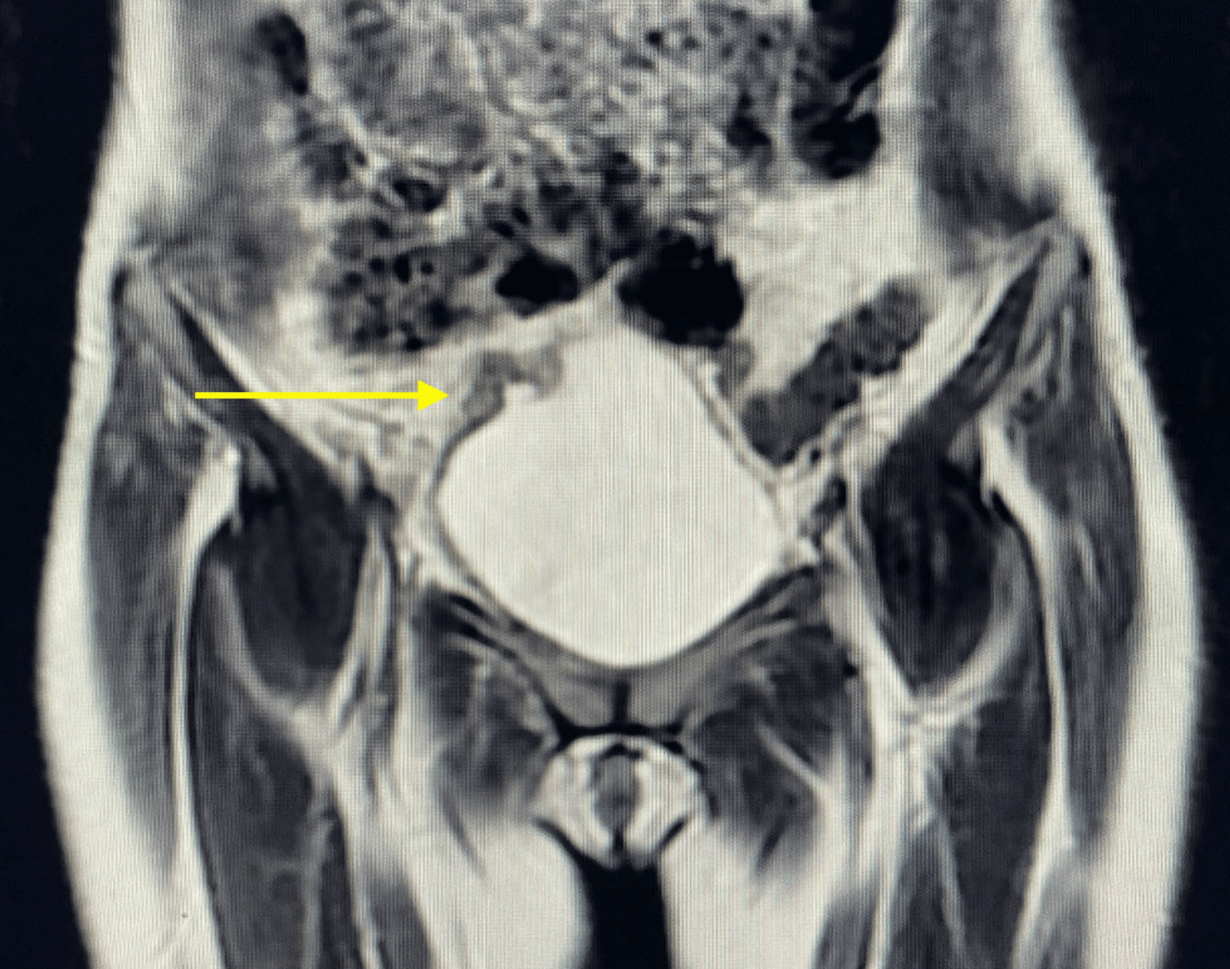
Coronal T2-weighted MRI demonstrating a large intravesical mass arising from the right lateral bladder wall. The lesion appears hyperintense relative to muscle, with well-defined borders and mass effect on the bladder lumen, consistent with bladder metastasis (yellow arrow).
